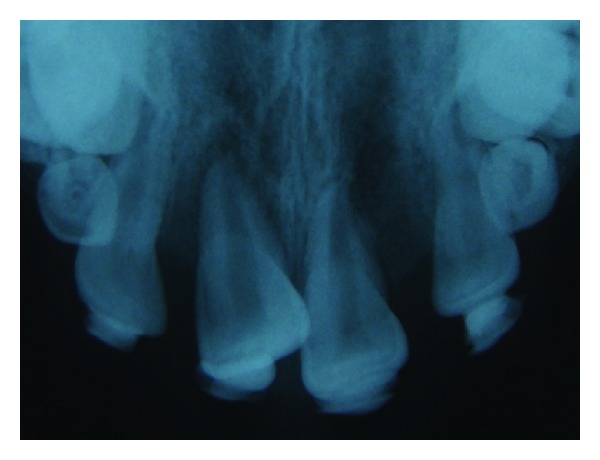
Radiographic view of the autotransplanted incisor at the 3rd week.
